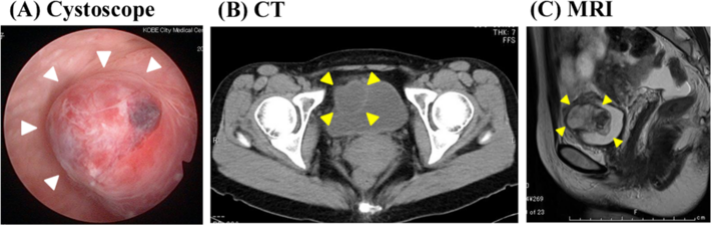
Cystoscopy, computed tomography, and magnetic resonance imaging findings. (A) Cystoscopy, (B) computed tomography, and (C) magnetic resonance imaging. White and yellow arrowheads indicate the submucosal tumor in the urinary bladder.
